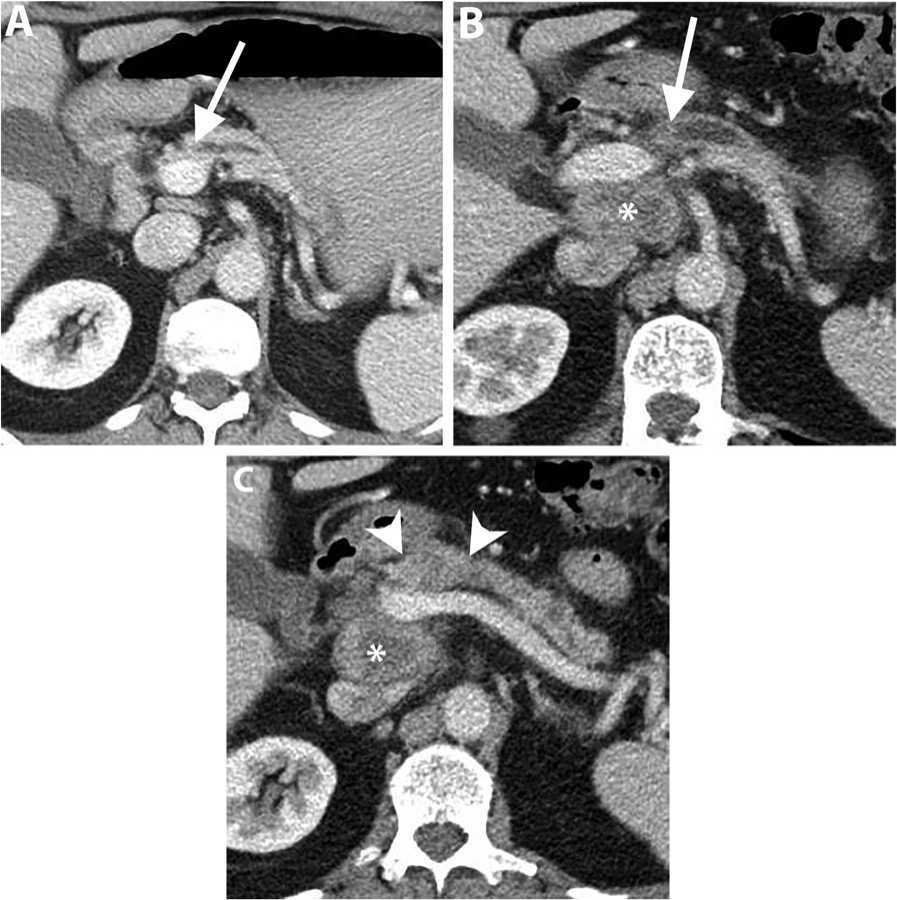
A 72-year-old man with PDAC. Axial portal venous phase CT image (a) shows MPD stricture (arrow) at the pancreatic body with upstream dilatation without visible obstructing mass. Axial portal venous phase CT images (b and c) obtained 6 months later show the progression of the MPD stricture (arrow) with worsened upstream ductal dilatation and pancreatic atrophy. There is a small hypoattenuating mass (arrowheads in c) associated with an enlarged necrotic metastatic portacaval lymph node (asterisk)
